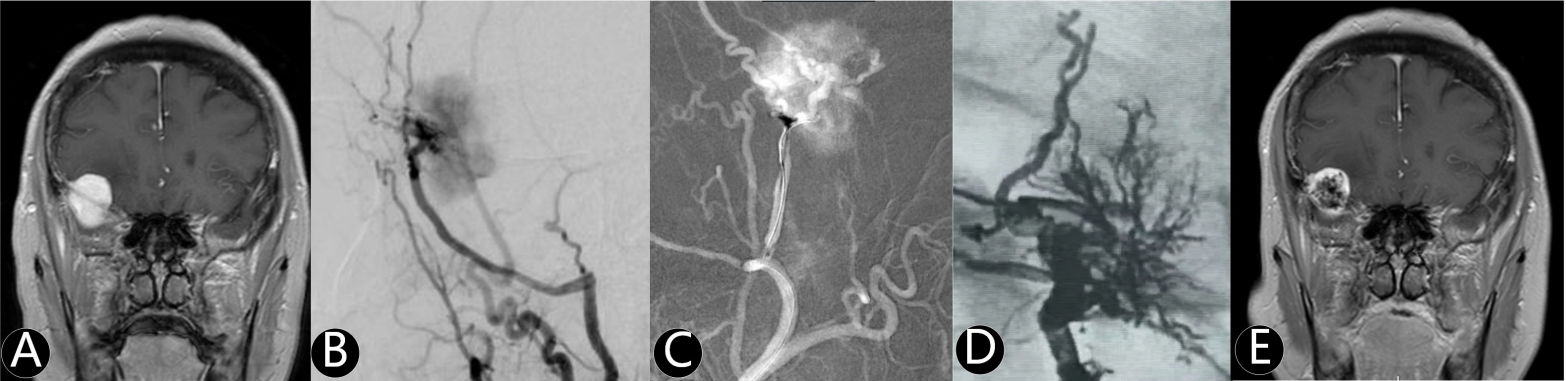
The patient was found right frontotemporal mass by accident. (A) Enhanced MR imaging showed right sphenoidal ridge mass with 28.7 cm3. (B) The middle meningeal artery was the main supply artery confirmed by cerebral angiography. (C) The microcatheter entered the supplying artery and Onyx 18 was injected into the tumor. (D) The total tumor (90%) was embolized without craniotomy. (E) The volume of tumor reduced at one year after simple embolization.
